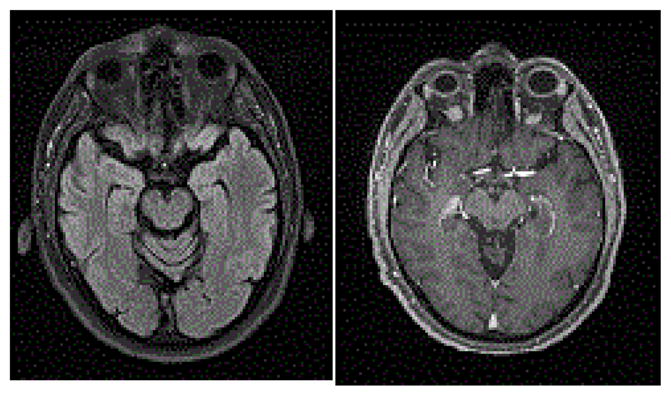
A. Resolution of periaqueductal hyperintensities, and B. mammillary bodies’ enhancement on a 3rd MRI conducted five weeks after admission. These figures document complete resolution of the abnormalities described on Figure 1.
